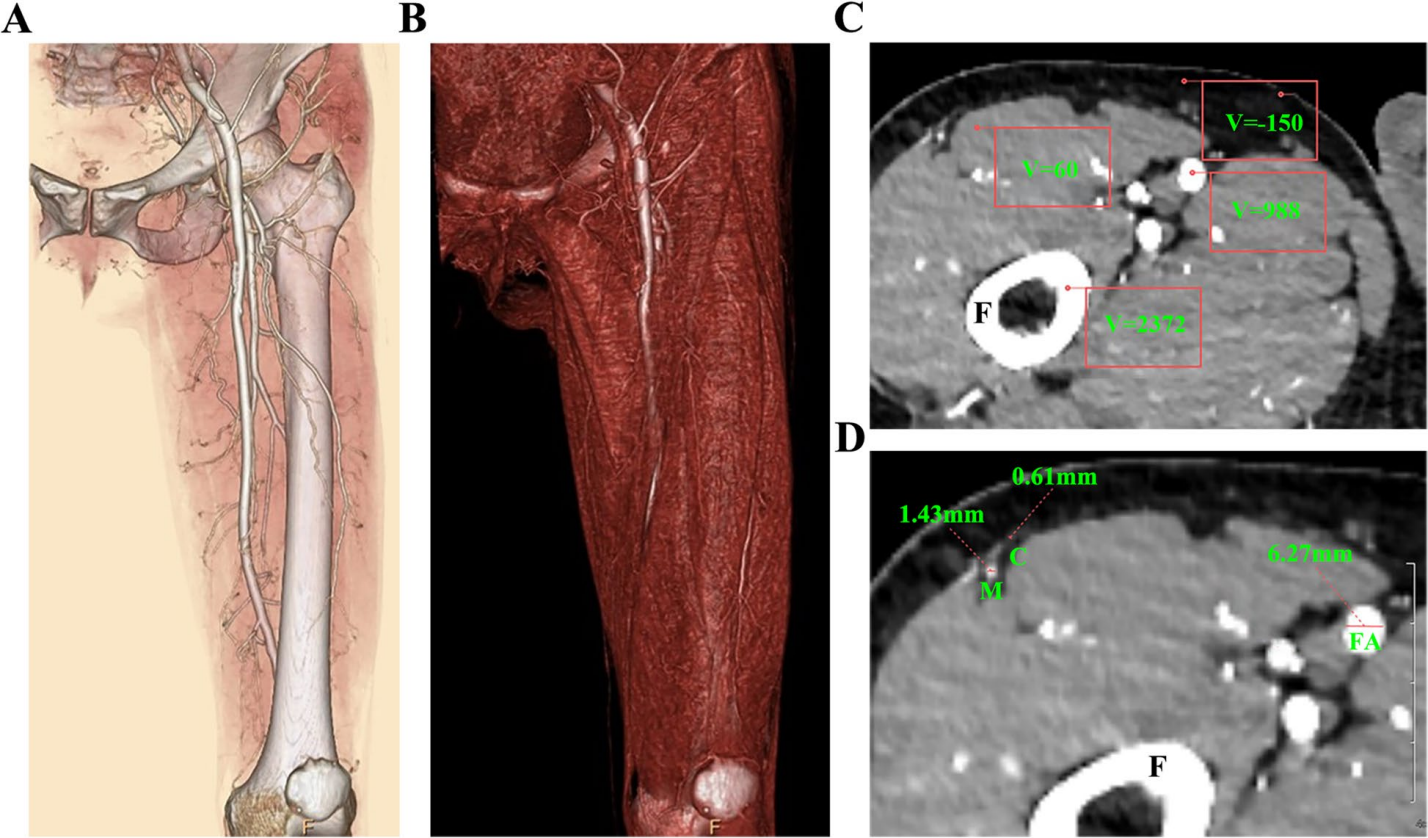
Three-dimensional reconstructed CTA images of the thigh. A Region of interest ranging from ASIS to patella was selected to reveal the whole view of thigh and ALTP. B Volume rendering of the muscles and artery, to display the perforators emerging from the deep fascia. C Different tissues possess different Hounsfield unit (HU) values in CT scan (Bone: 2000-2500HU, Artery: 800-1200HU, Muscle: 50-80HU, Fat: under -140HU). D The diameters of femoral artery, descending branch, perforators, and their cutaneous branches
